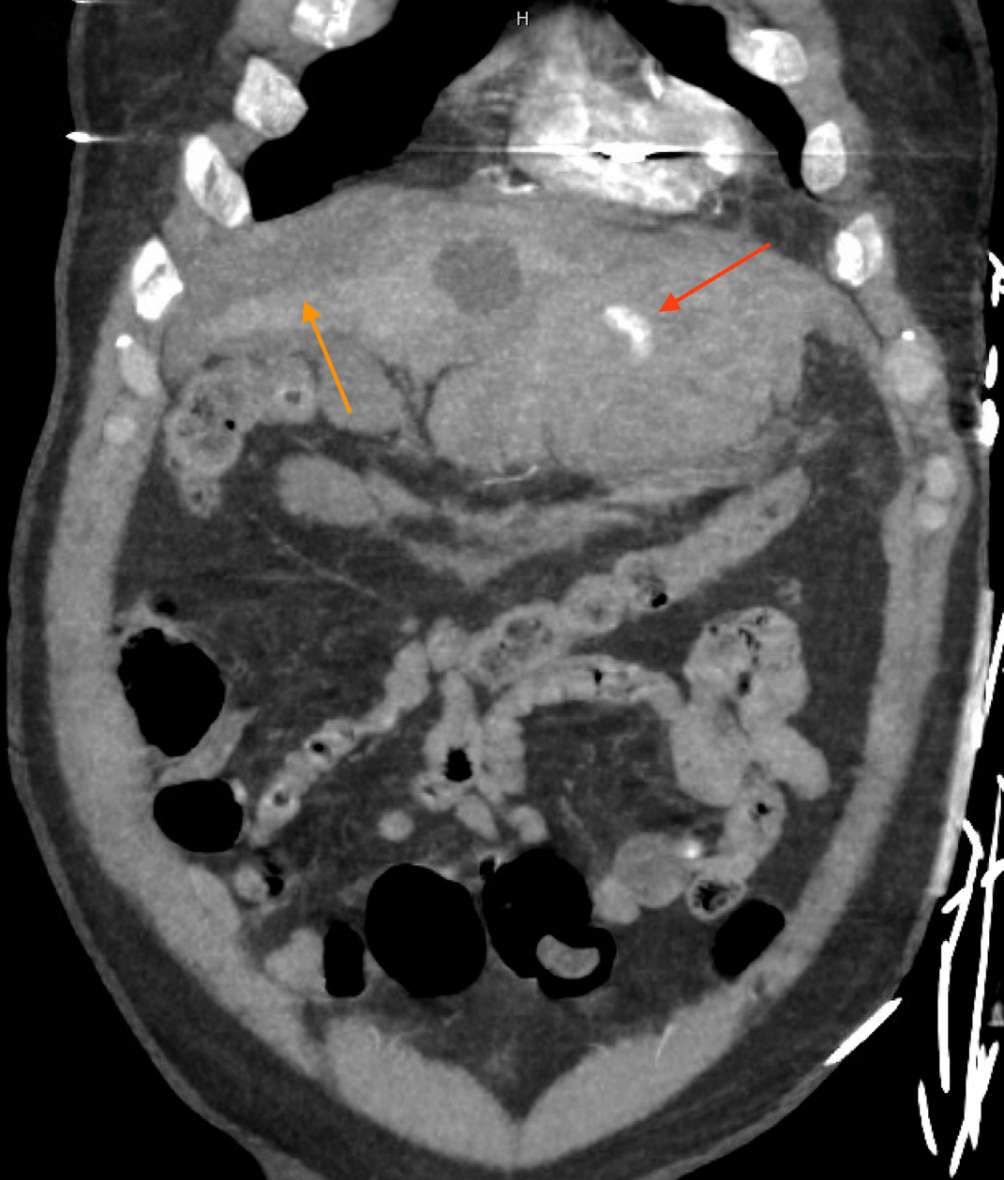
Computed Tomographic Angiography of the Abdomen and Pelvis With Left Internal Mammary Artery Bleed Coronal view depicting active arterial contrast extravasation and bleed in the epigastric region of the peritoneum (red arrow), surrounded by hemoperitoneum (orange arrow).
